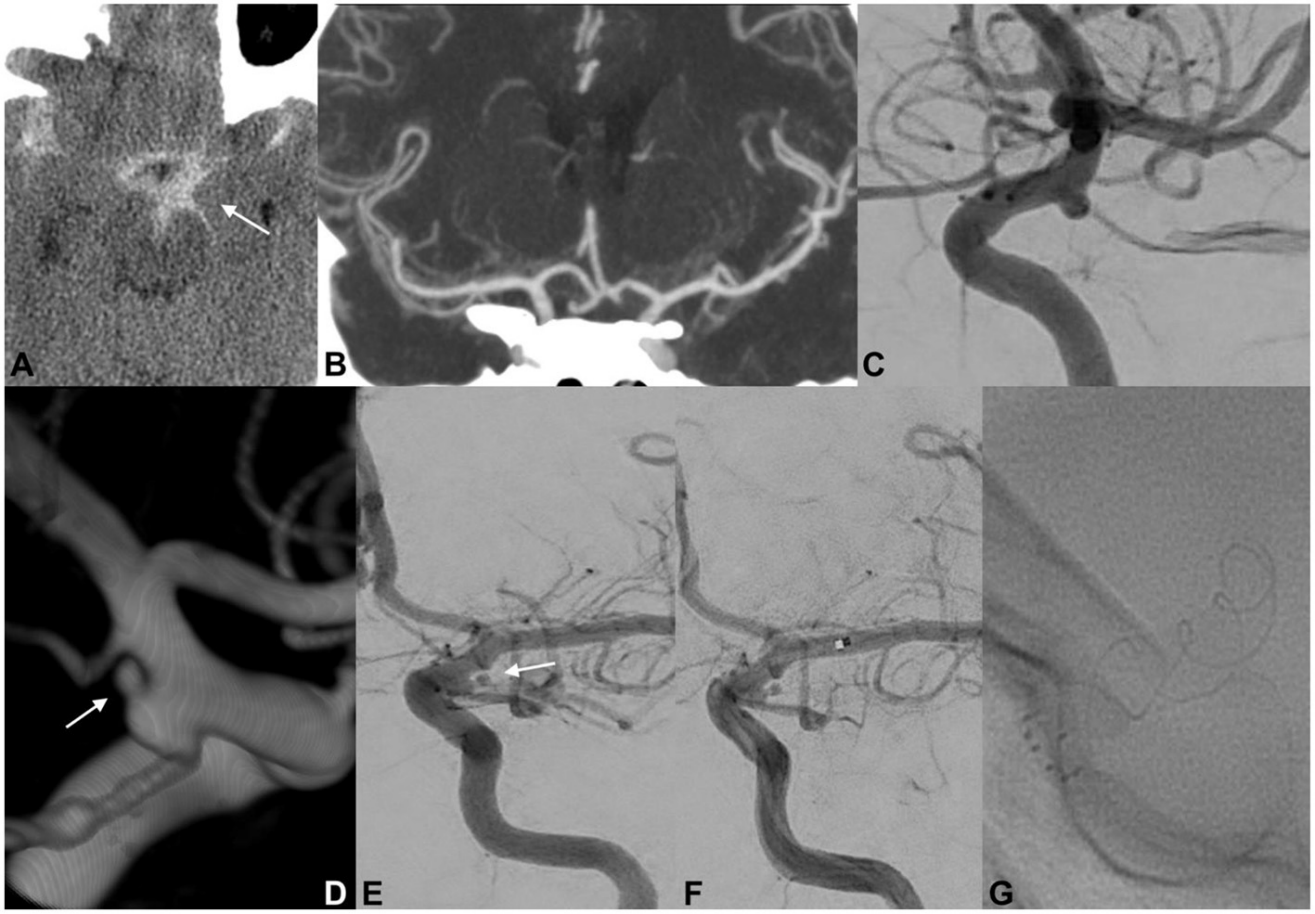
EVT of a ruptured PcomA ID. An initial CT scan demonstrated the presence of SAH predominantly distributed across the left basal cisterns (A; white arrow). Cranial CTA did not document any cerebrovascular findings that could have caused the SAH (B). At 6 h after onset, catheter angiography confirmed the absence of a ruptured aneurysm but revealed a typical ID at the origin of the left PcomA (C). Delayed 3DRA/DSA (D,E; white arrows) 7 days after onset identified a possible rupture bleb on the lateral side of the left PcomA ID. Endovascular coil embolization of the ruptured bleb was unsuccessful, and a p64 FD was carefully deployed across the C7 segment of the left ICA (F,G). Contrast stagnation inside the ruptured ID bleb was seen on the delayed angiographic phase (not provided).
